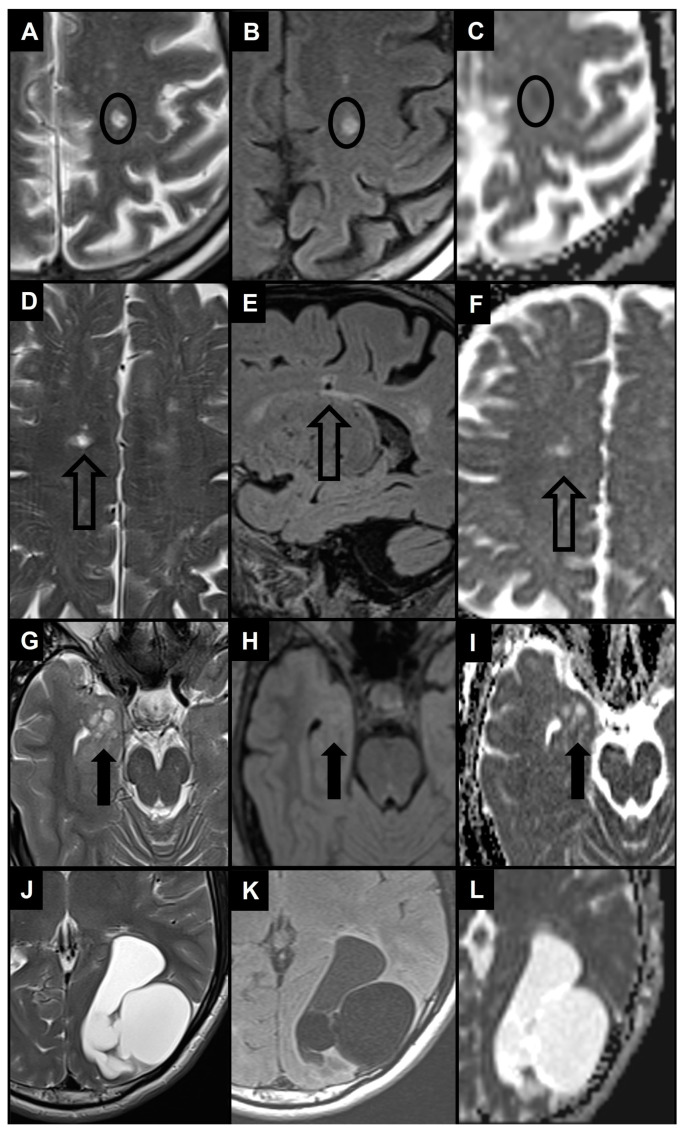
The 1.5 T magnetic resonance images show the main differential diagnosis for enlarged PVS in the context of cerebral small vessel disease (A–F) and 2 examples of differential diagnosis for giant PVS (G–L) using T2 turbo spin echo images (A,D,G,J), FLAIR images (B,E,H,K) and apparent diffusion coefficient images (C,F,I,L). (A,B) Patient with a small area of altered signal in the left centrum semiovale (black circle) characterized by hyperintensity in T2 and FLAIR images and diffusion restriction, therefore compatible with a recent subcortical infarction. (D–F) Patient with a small area of altered signal in the right centrum semiovale (empty black arrow) characterized by hyperintensity in the T2 image, hyperintense rim in the FLAIR image, and no diffusion restriction, therefore compatible with a lacune. Note also the white matter hyperintensities in centrum semiovale bilaterally. (G–I) Patient with a dysembryoplastic neuroepithelial tumor in the right medial temporal lobe (thick black arrow), with a distinct “bubbly” cystic appearance associated with T2/FLAIR signal abnormality and no diffusion restriction. (J–L) Patient with a left parieto-occipital neurenteric cyst characterized by a slightly hyperintense content in FLAIR images and no diffusion restriction.
